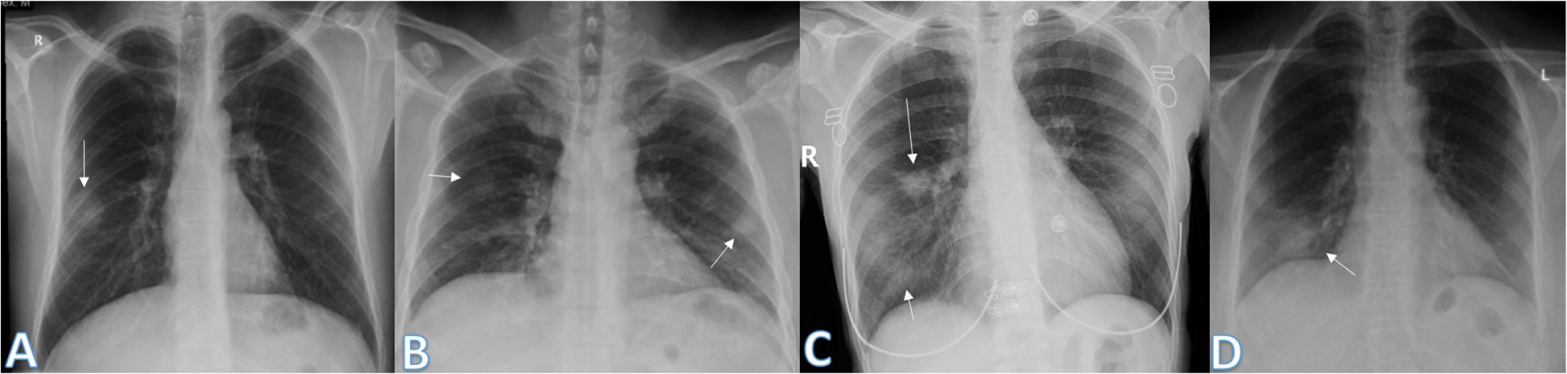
Four different patients with positive COVID-19. a 32-year male was complaining of cough. Initial chest X-ray showed a well-defined solitary pulmonary nodule at the lateral aspect of the right middle lung zone (arrow). The total severity score was 1. b 49-year male was complaining of fever. Initial chest X-ray showed two small well-defined pulmonary nodules at both middle lung zones (arrows). The severity score was 1 for each lung, so TSS was 2. c 36-year female was complaining of sore throat. Initial chest X-ray showed right perihilar fairly defined pulmonary nodule (long arrow) and right lower zonal ill-defined ground glass opacity (short arrow). The total severity score was 2. d 52-year female patient presented with cough, showed a well-defined solitary pulmonary nodule at the right lower lung zone (arrow). The total severity score was 1
